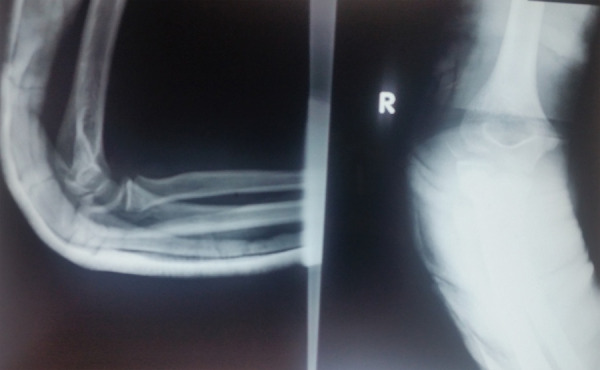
Post reduction X-ray of same elbow with application of posterior slab.
